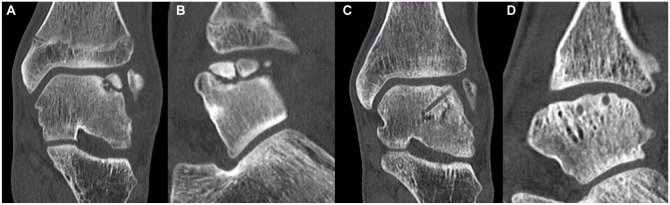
Case 3. Pre-operative CT with coronal (A) and sagittal (B) views, showing an anterolateral osteochondral fragment. The fragment was comprised of 2 smaller fragments and was likely traumatized previously. The lesion measured anteriorposteriorly: 25 mm, medial-laterally: 11 mm, and depth: 8 mm. One-year post-operative CT with coronal (C) and sagittal (D) views, showing the union of the fragment and a good bony integration. It should be mentioned that the radiolucency in the talus is the bio-absorbable screw.
